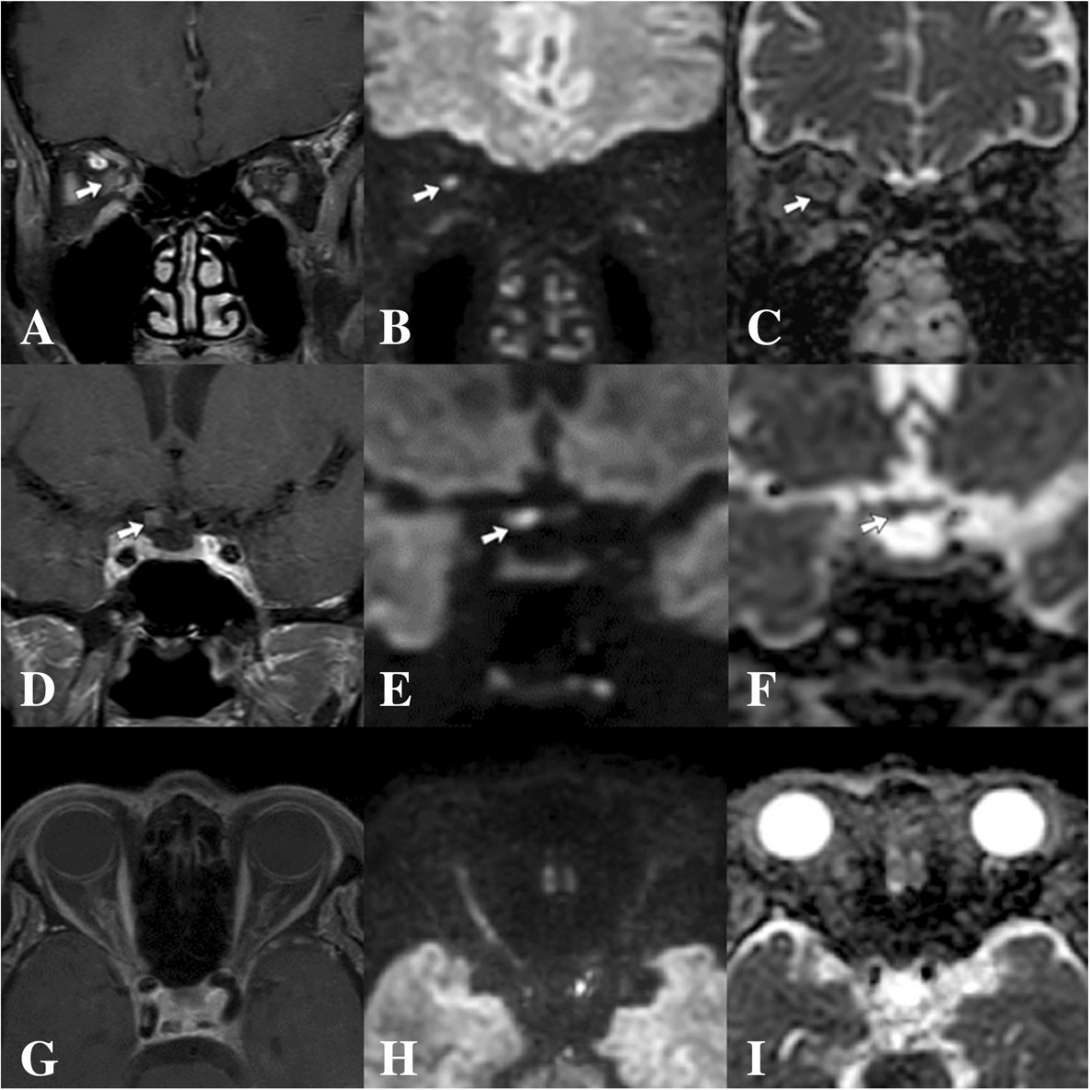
Magnetic resonance imaging of the orbit with diffusion weighted imaging (DWI) and an apparent diffusion coefficient (ADC). Coronal T1 weighted with a gadolinium injection (a) shows enhancement of the right intraorbital optic nerve (arrow) along with restricted diffusion (arrow) on DWI (b), which was confirmed by the low signal (arrow) on the ADC map (c). Coronal T1 weighted with a gadolinium injection (d) shows enhancement of the right prechiasmatic optic nerve (arrow) along with restricted diffusion (arrow) on DWI (e), which was confirmed by the low signal (arrow) on the ADC map (f). Axial T1 weighted with a gadolinium injection (g) shows enhancement of the right optic nerve along with restricted diffusion on DWI (h), which was confirmed by the low signal on the ADC map (i)
